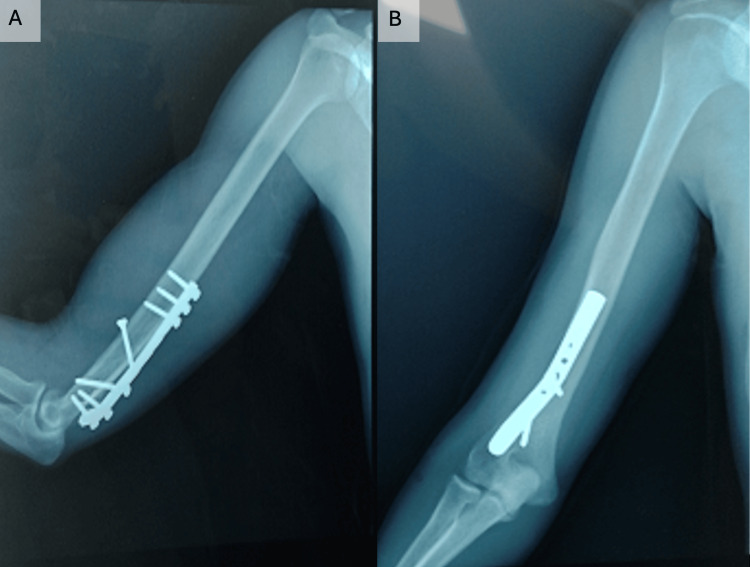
One month after fixing the fracture of the distal end of the humeral shaft with a screw plate, an X-ray was taken to monitor/control the healing process. (A) Profile impact, (B) front impact
